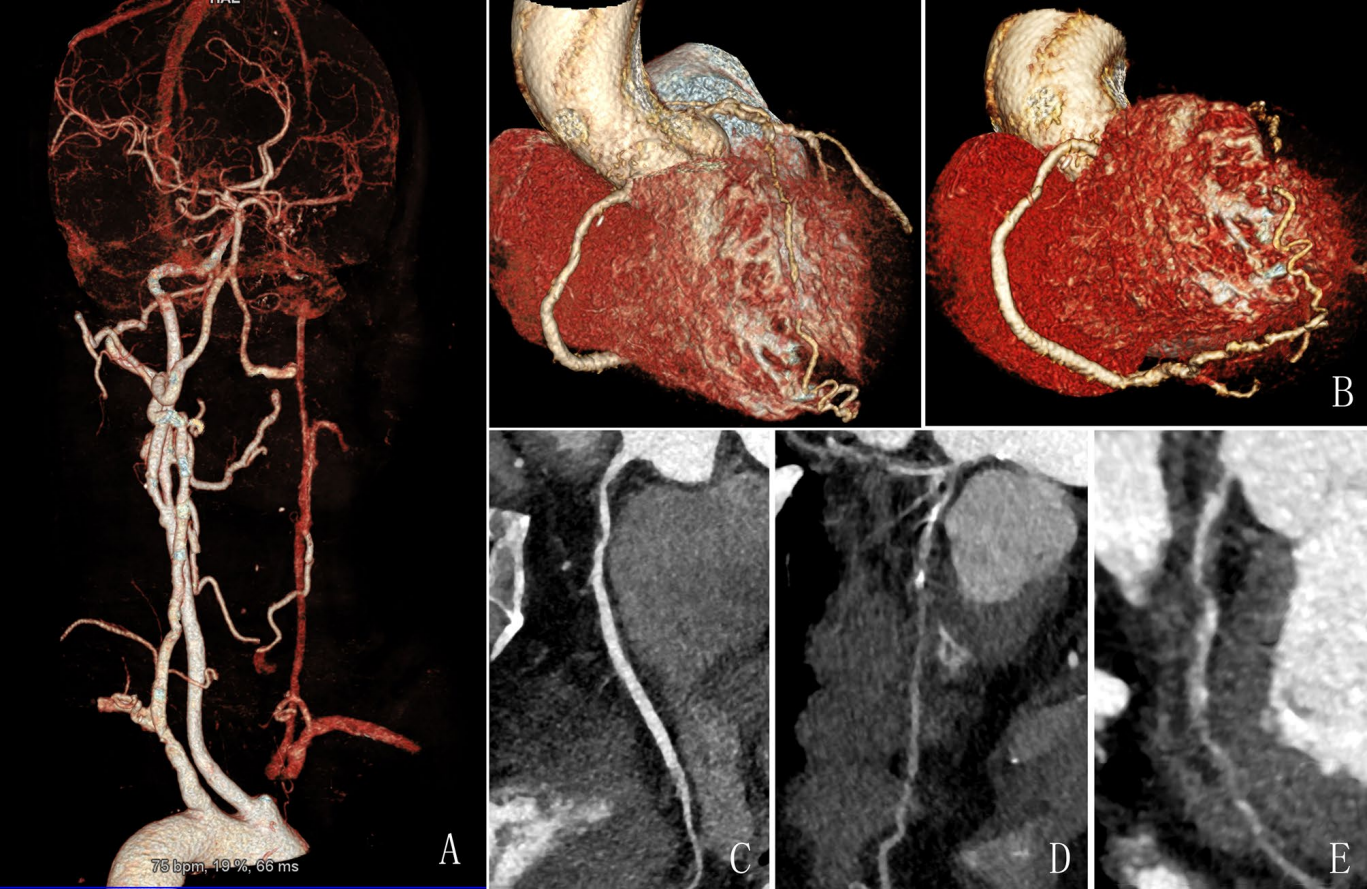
A 50-year-old male patient with progressive leg weakness, numbness, and dizziness for 2 days. ICCC-CTA detected coexisting plaques in the carotid [total occlusion in the proximal brachiocephalic trunk (A)] and coronary arteries [stenosis in RCA (C), LAD (B and D), and LCx (E) arteries]. ICCC-CTA, Integrated coronary–carotid–cerebral computed tomography angiography; LAD, left anterior descending; LCx, left circumflex; RCA, right coronary artery; VR, volume rendering
